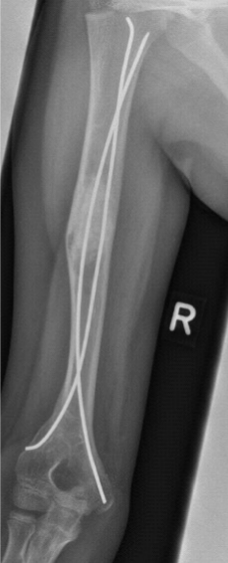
Failed Consolidation after treatment with nailing alone. Elastic stable intramedullary Nailing in combination with Orthoss^® ^and GPS^® ^after earlier failed treatment. Radiograph three months following the initial GPS^®^/Orthoss^® ^treatment resulting in bone mineralization.
